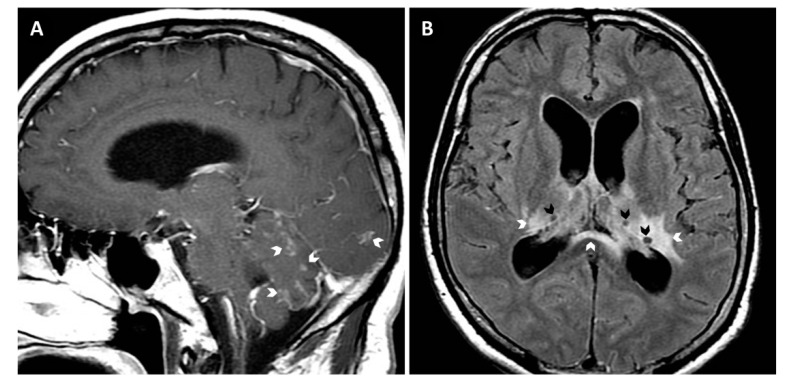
Brain MRI images (A, sagittal T1Gd and B, axial T2-FLAIR [fluid attenuated inversion recovery] showing generalized leptomeningeal enhancement (arrows in A), ganglionar and periventricular edema (white arrows in B), cystic lesions suggestive of gelatinous pseudocysts (black arrows in B), and enlarged ventricles compatible with communicating hydrocephalus.
